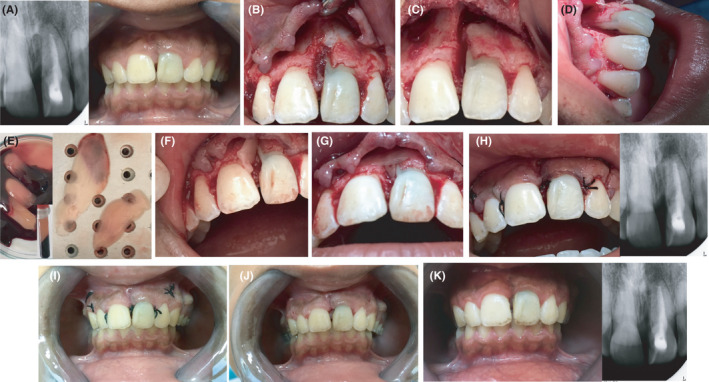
Re‐intervention surgery after 4 years to remove retained apex of the supernumerary tooth and re‐plane the resected mesial surface. (A) Immediate pre‐operative periapical radiograph showing a periapical radiolucent lesion and retained apex of supernumerary tooth (left) and clinical photograph (right); (B) Following flap reflection revealing long vertical defect on mesial surface of maxillary left central incisor; (C, D) Creation of a vertical groove reaching the retained apical segment of the supernumerary tooth; (E) Preparation of Platelet‐Rich Fibrin (PRF) membranes; (F) Planing of root surface and placement of PRF membrane in the vertical groove; (G) Placement of second PRF membrane underneath the flap prior to repositioning; (H) Final clinical image after suturing (left) and post‐operative periapical radiograph (right) showing resection of root end of maxillary left central incisor and shaving off of retained apex; (I) One week after the re‐intervention surgery; clinical image after removal of sutures; (J) clinical image 2.5 months after re‐intervention surgery; (K) Clinical image and periapical radiograph one year after re‐intervention surgery showing periapical healing and deposition of new bone
